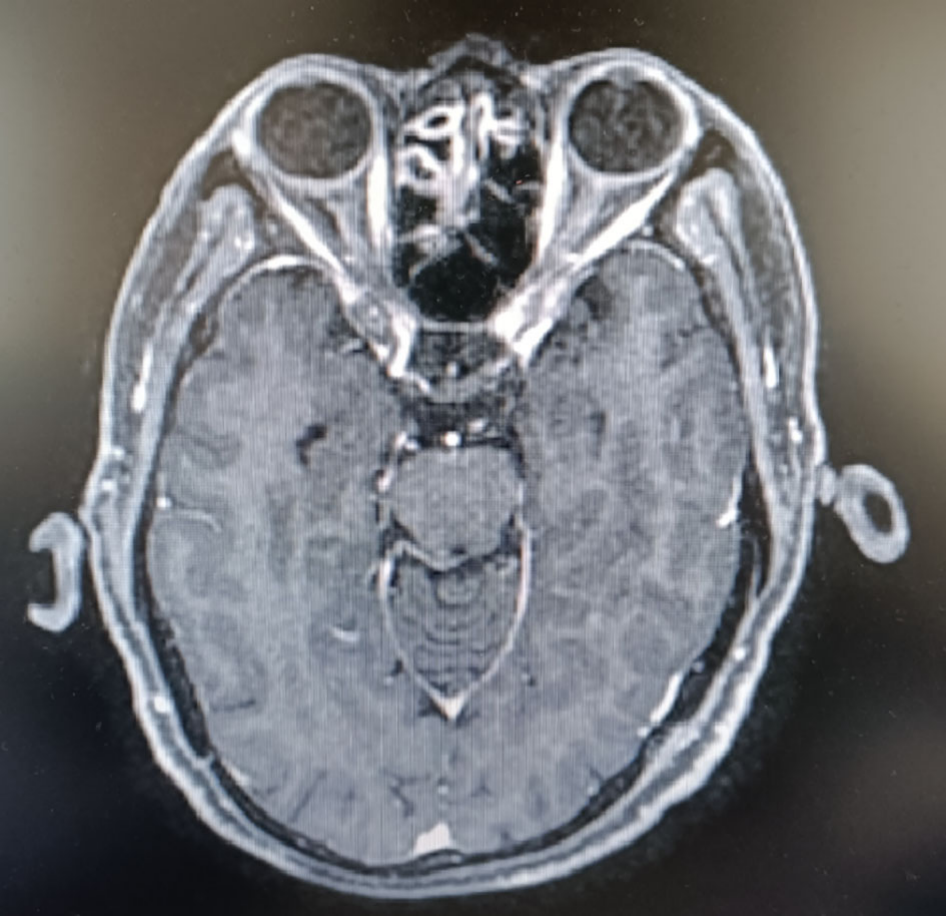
The baseline brain MRI of the patient.
